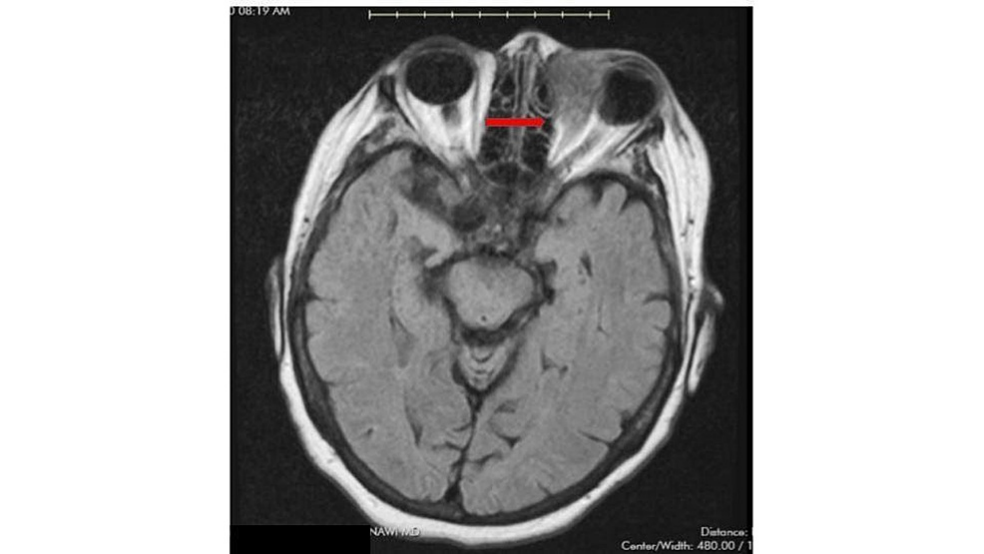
MRI revealing heterogeneously enhancing extraconal mass within left orbit infiltrating into the medial canthus region (red arrow).
